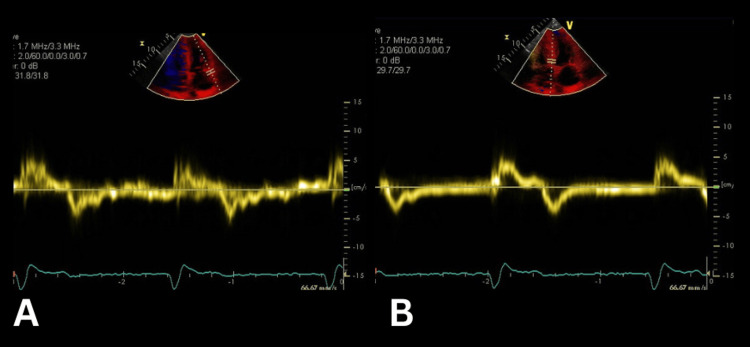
Tissue Doppler imaging (TDI) during transthoracic echocardiogram (TTE) (A) Lateral S' (mitral annulus) mean velocity of 4.7 m/second (normal >5 m/second). (B) Septal peak E' velocity of 5.1 m/second (normal >6 m/second).
